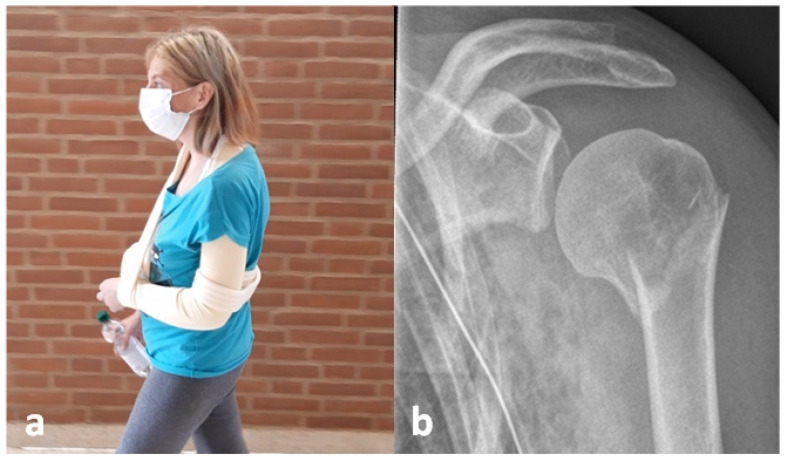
The patient was, several days later, able to walk independently (a). An anteroposterior X-ray control before chest tube removal in an upright standing position indicates a nearly anatomic HSA (b).
